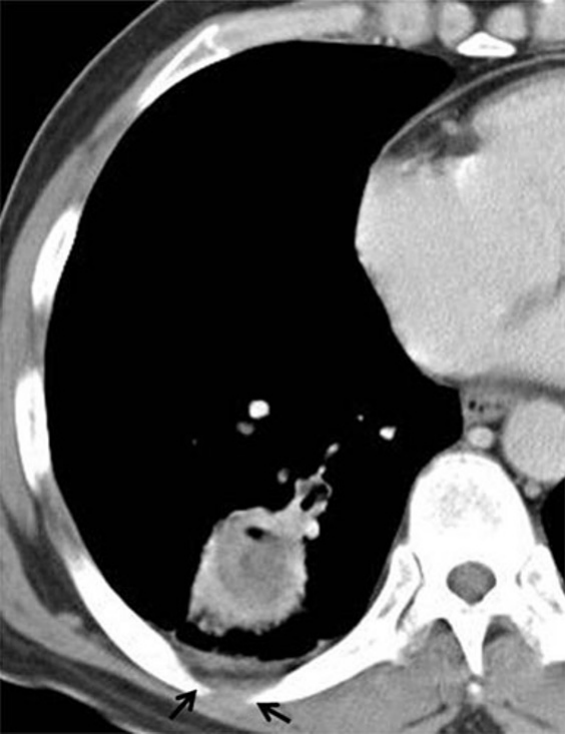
Typical parenchymal actinomycosis in a 58-year-old man with haemoptysis for a month. The CT scan in the mediastinal window setting shows a 3-cm mass-like consolidation with central, low-density peripheral rim enhancement in the right lower lobe and adjacent pleural thickening with subpleural fat infiltration (*arrow*)
